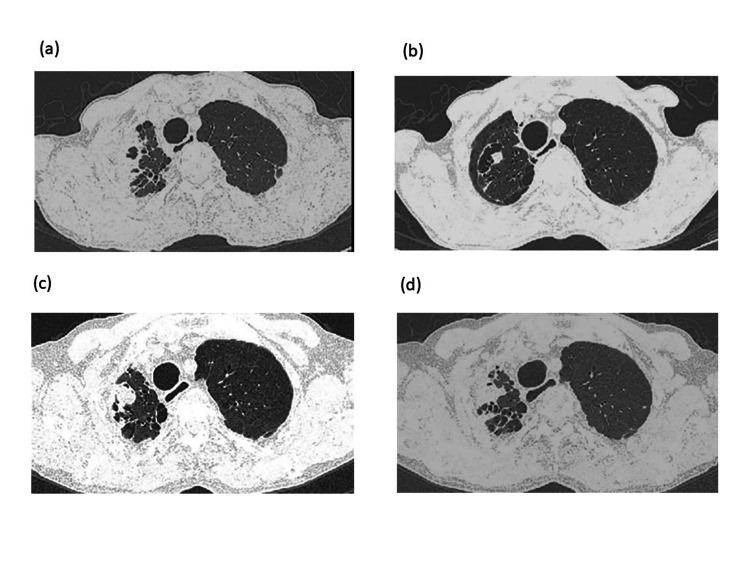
Chest CT. The CT scan reveals a cavity and pleural thickening of the right upper lung on day 1 (a), the disappearance of pleural thickening and residual cavity with a fungus ball lesion one month after receiving OCS (b), fungus ball lesion progression of the right lung while tapering OCS (c), and collapsed fungus ball lesion during mepolizumab administration (d). CT: computed tomography; OCS: oral corticosteroids.
